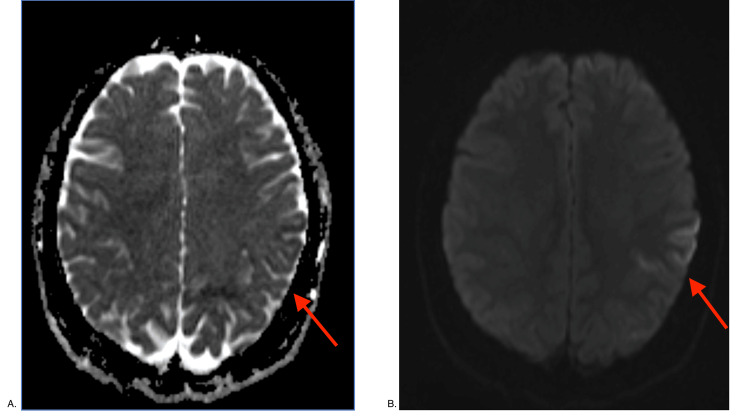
MRI of the brain without contrast (2A) that shows the restricted diffusion along the left frontoparietal cortical region, with the corresponding hypointensity on apparent diffusion coefficient (ADC), compatible with sequelae to acute ischemic infarction in diffusion-weighted imaging (DWI) in 2B (arrows).
